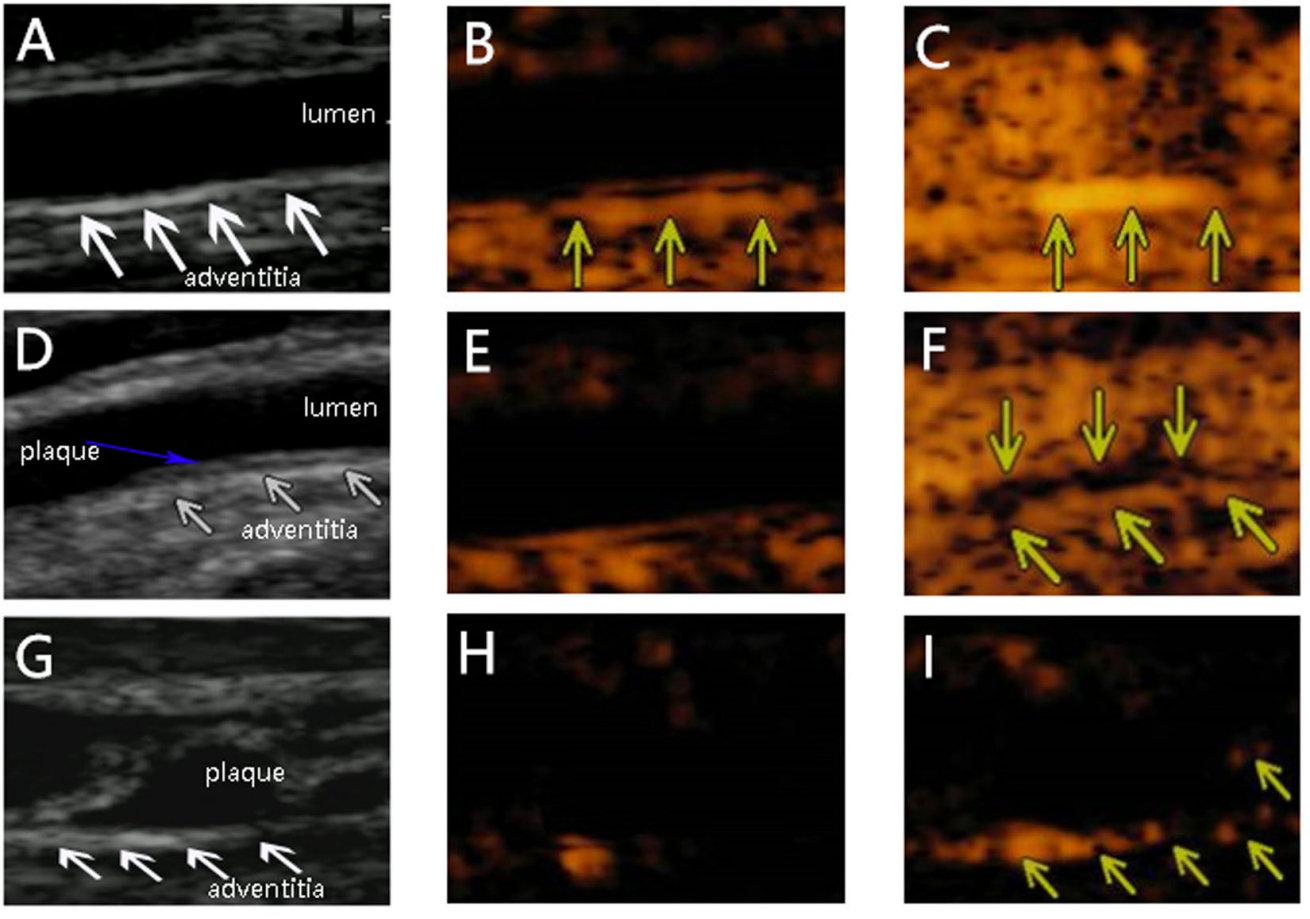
Two-dimensional ultrasound and contrast ultrasound images of the carotid before and after contrast injection. Two-dimensional ultrasound image of the carotid artery clearly shows the adventitia interface and lumen (A). The carotid artery lumen was dark before contrast injection, and the outer membrane is clearly visible as indicated by the yellow arrows (B). The carotid lumen became immediately visible, and the adventitial also showed enhanced signal after contrast injection, as indicated by the yellow arrows (C). There was a small plaque on the wall of the deep region as indicated by the blue arrows (D). The carotid artery lumen was dark before contrast injection (E). The plaque was a little filling defect, and the outer membrane was visible with more adventitial contrast as indicated by the arrows (F). There was a large mixed echo plaque almost filling the lumen (G). Before injection of contrast, the carotid artery lumen was dark (H). After contrast injection, the outer membrane was obviously enhanced and much more adventitial contrast was visible. There is contrast enhancement inside the plaque as indicated by the yellow arrows (I).
